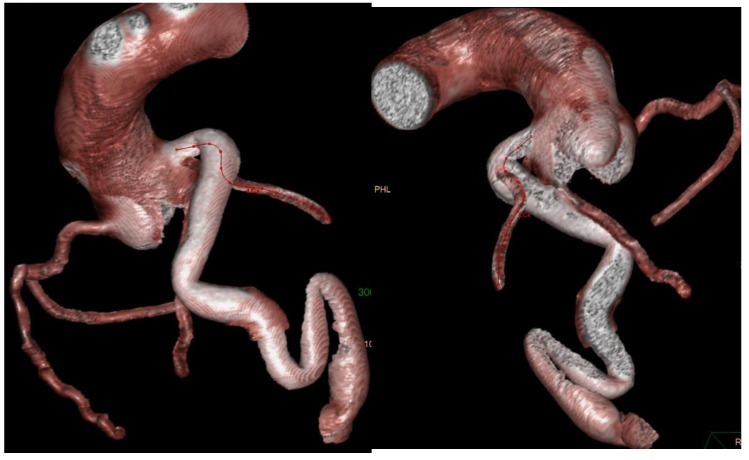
CT angiography focusing on coronary artery and fistula.
